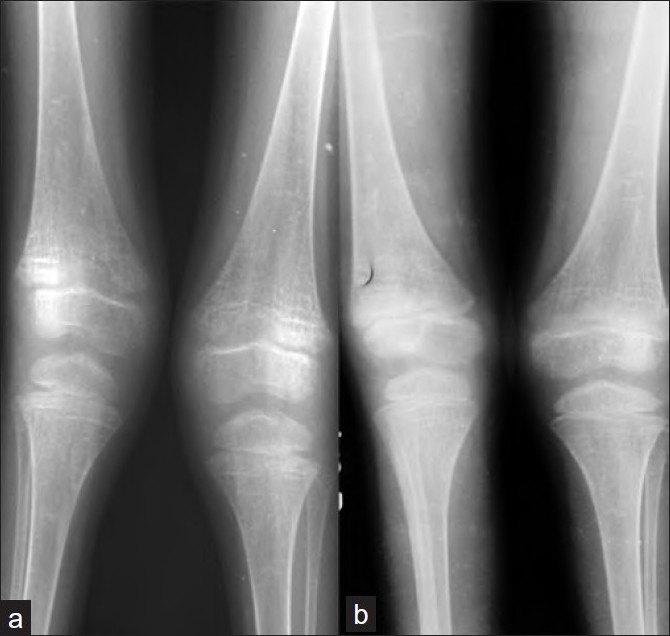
(a) Anteroposterior radiographs of both knee joints showing decreased bone density and trabeculations. (b) follow up radiograph at one year shows bone density has improved and trabeculations are less prominent
